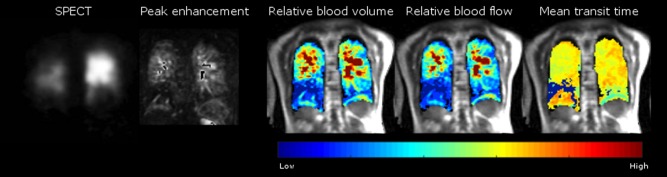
Matched slices from a single patient with CTEPH showing the SPECT and the peak enhancement image from a DCE perfusion MRI scan used clinically, alongside semiquantitative perfusion maps (pulmonary blood volume, flow, and mean transit time) from a DCE perfusion MRI.
